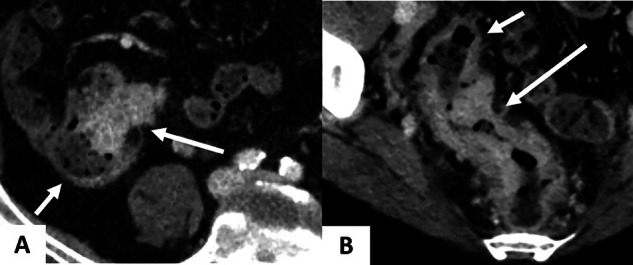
72-year-old (A) and 67-year-old (B) males with biopsy-proven adenocarcinoma. DS-DECT (Siemens Force) in axial section at VP. A The 40 keV VMI image shows a marked enhancement of the primary tumor (long arrow) compared with the adjacent normal wall. IC was 2.1 mg/mL. B The 40 keV VMI image also shows a slightly lower enhancement of the primary tumor (long arrow) compared to the adjacent normal wall (short arrow). IC was 1.6 mg/mL. A corresponds to well-differentiated tumors, whereas B corresponds to a poorly differentiated tumor. DS-DECT, dual-source dual-energy CT; IC, iodine concentration; VMI, virtual monoenergetic images
